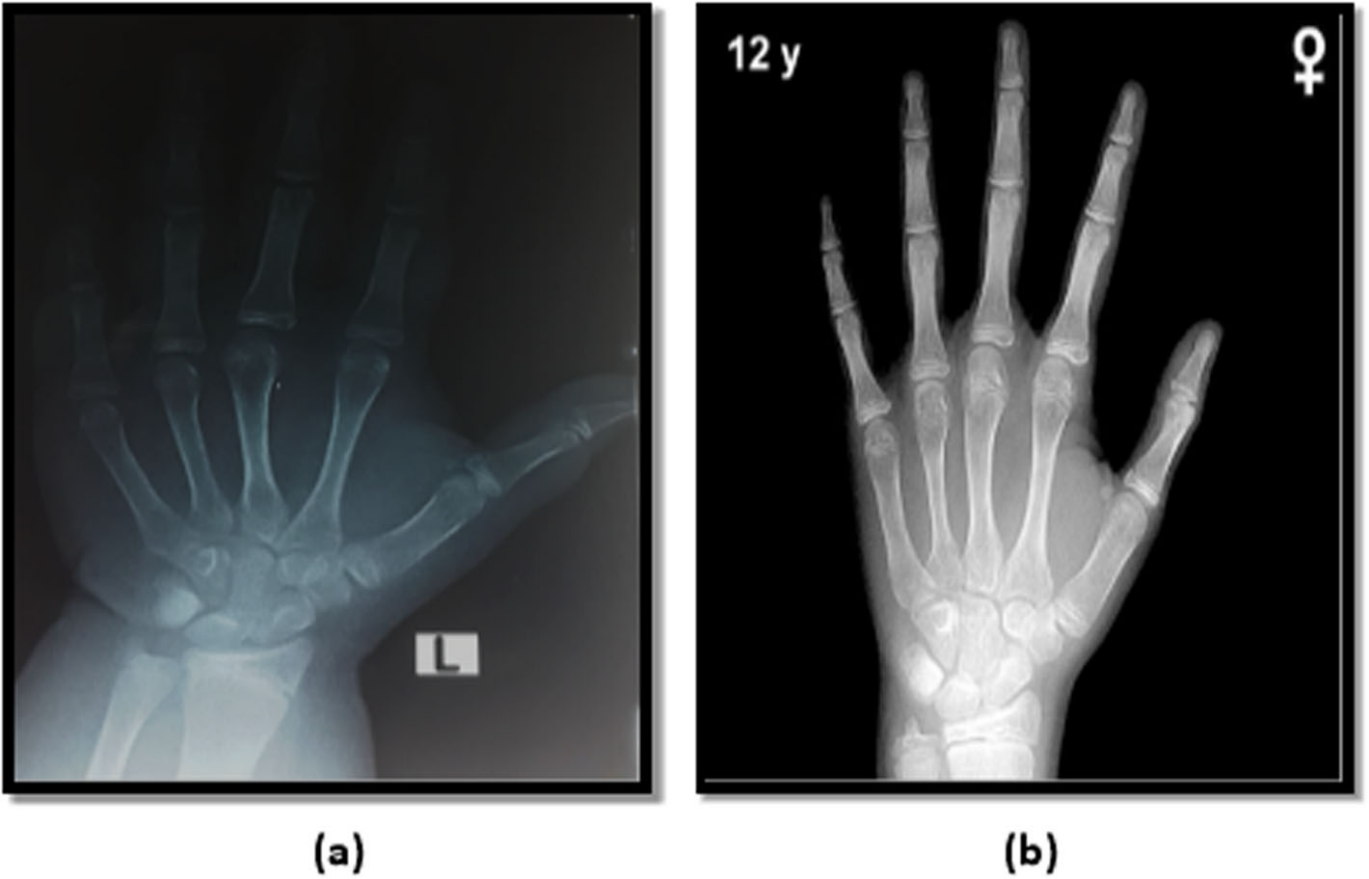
a Radiograph of the left hand obtained in an 11.4-year-old Egyptian girl. b Greulich and Pyle method atlas image showing advanced bone age (12 years old)
